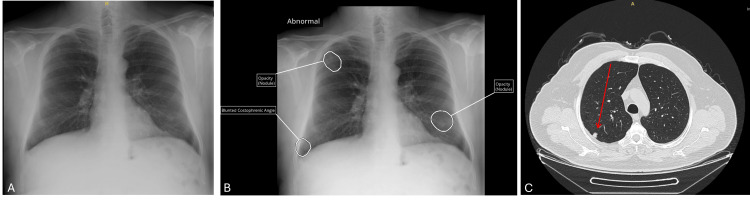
Chest X-ray and CT images of patient 1 A: Chest X-ray image without AI; B: Interpretation of chest X-ray with AI (suspicious nodules marked); C: Computed tomography (CT) image of the thorax (arrows show nodules)
